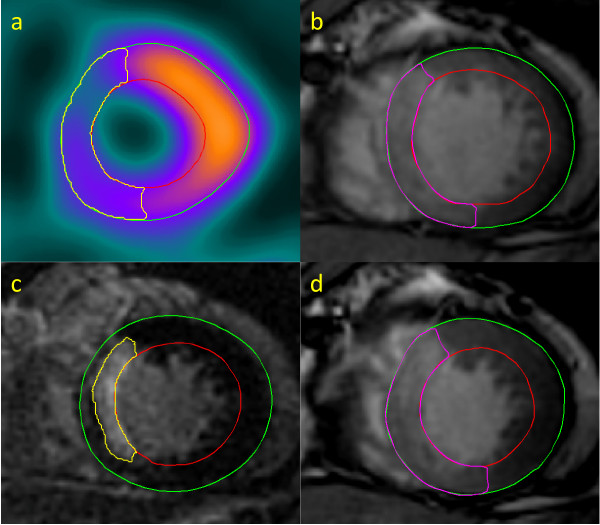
Anterior STEMI. Corresponding left ventricular short axis views from a patient with anterior myocardial STEMI. MaR determined by (a) myocardial perfusion SPECT, (b) gadolinium enhanced SSFP at end-diastole, (c) infarct size images with LGE and (d) gadolinium enhanced SSFP at end-systole. It is clearly seen that the region of gadolinium enhancement does not correspond in size or endocardial extent to the region of myocardium at risk either by SSFP cines or myocardial SPECT.
